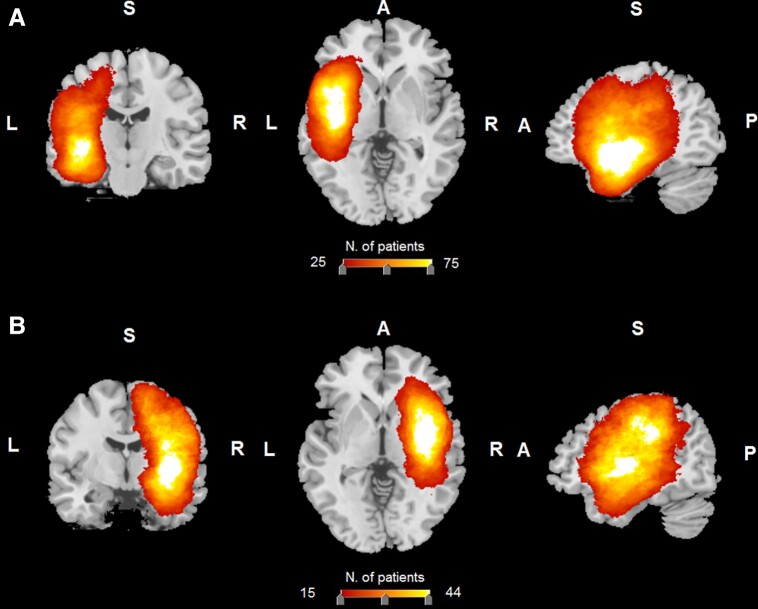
Brain areas maximally invaded by the tumour in the whole patients’ sample (*N* = 743 lesion volumes). Brain areas maximally invaded by the tumour in the whole patients’ sample (*N* = 743 lesion volumes) are shown in the overlays of lesion volumes with lesions to the (A) left hemisphere, and (B) right hemisphere on coronal, sagittal, and axial sections of the T1-weighted MRI template a standard reference for brain MRI. The legend shows the colour corresponding to the different frequencies of the participants’ lesion overlays, from red, least frequent, to white, most frequent.
